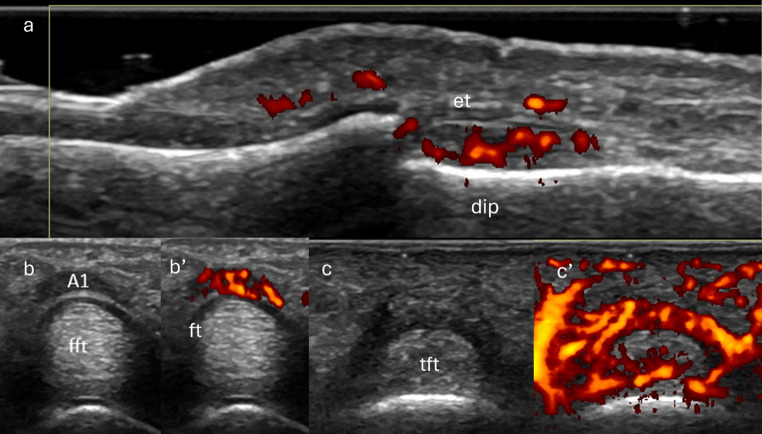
Ultrasound detection of subclinical inflammation in patients with psoriasis at risk of psoriatic arthritis. Legend. (a) Synovitis in the distal interphalangeal joint (DIP) joint of the finger with synovial hypertrophy and power Doppler signal (dorsal longitudinal scan); the image also shows enthesitis of the tendon insertion into the distal phalanx with entheseal power Doppler signal. (b) Grey-scale transverse volar scan of the A1 pulley demonstrates severe thickening with hypoechoic appearance, accompanied by Power Doppler (b’) signal. (c) Grey-scale image of tenosynovitis in the flexor tendons of the 2nd toe with synovial hypertrophy and active inflammation (the latter demonstrated by the presence of power Doppler signal) (c’) (volar transverse scan). Acronyms: dip: distal interphalangeal; et: extensor tendon; fft: finger flexor tendons; tft: toe flexor tendons
